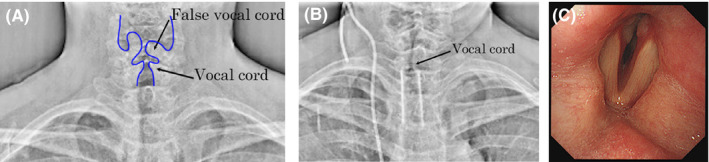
Dynamic digital radiography (DDR) during deep inspiration on day one (A) and day nine (B) and bronchoscopy during phonation on day nine (C) in Case 1. DDR on day one (A) shows paramedian fixation of the left vocal cord, and the bilateral vocal cords on day nine (B), as confirmed using bronchoscopy on the same day (C).
